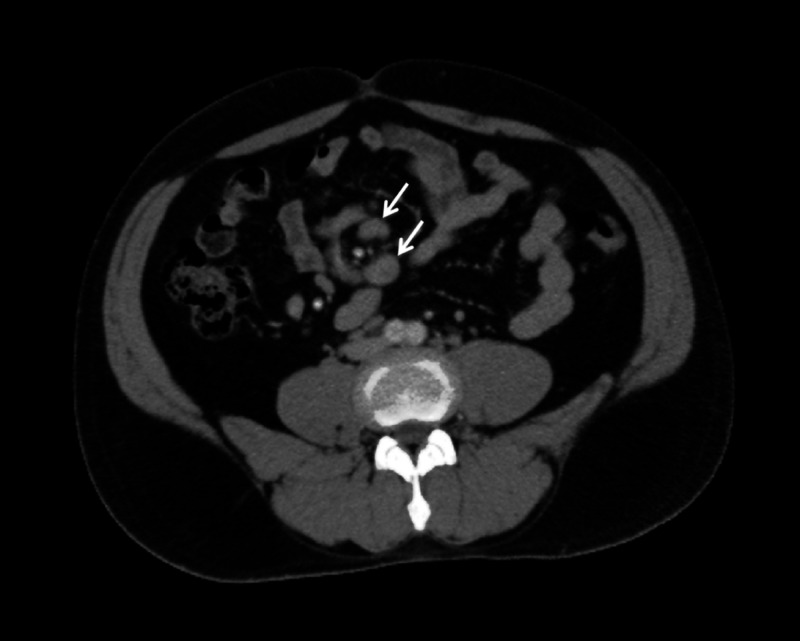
Computed tomography post-intravenous contrast axial section (soft tissue window) at the level of aortic bifurcation showing enlarged mesenteric lymph nodes (arrows).
